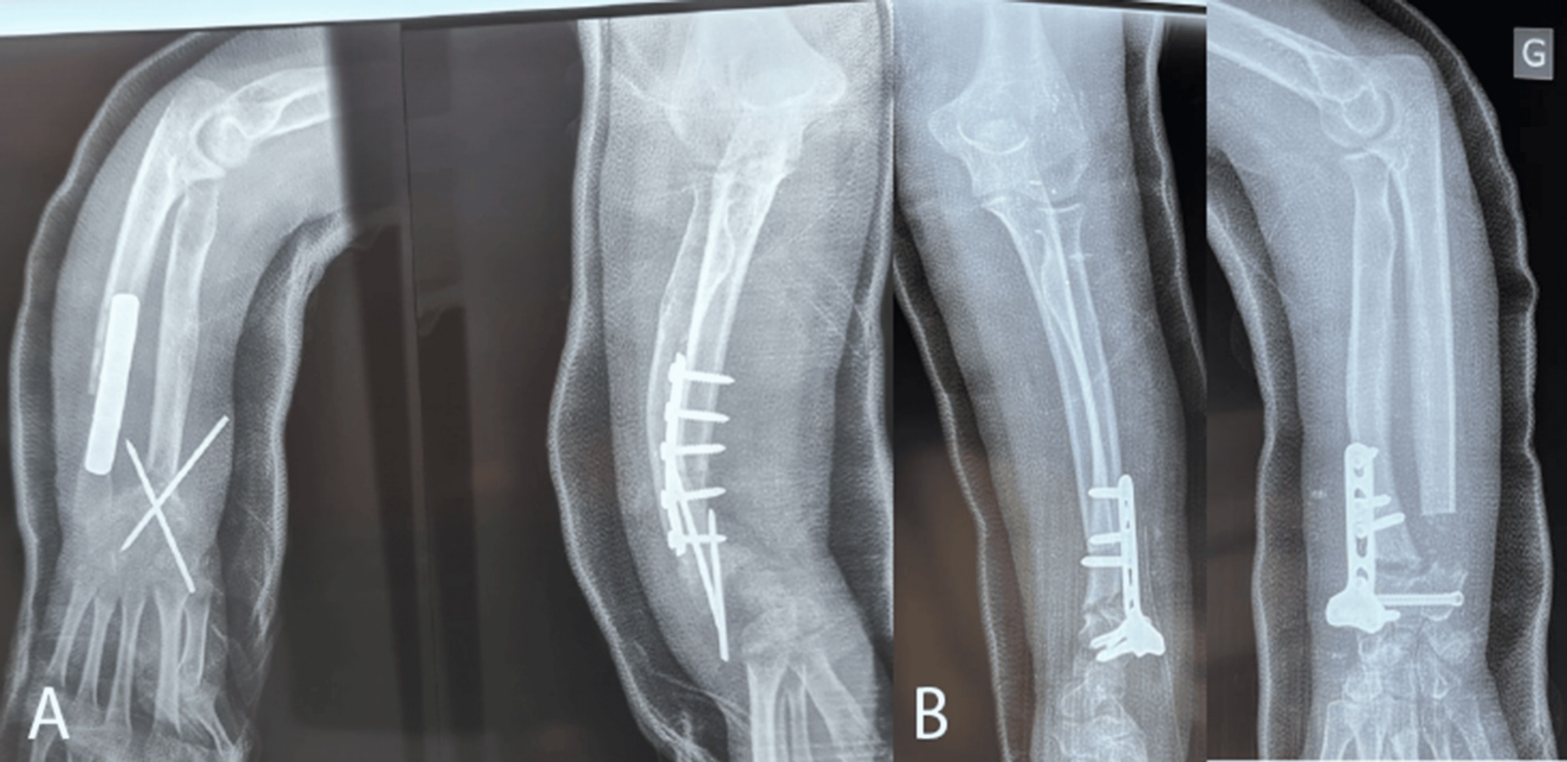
Postoperative radiographs of the forearms in the same patient: (A) right forearm and (B) left forearm
